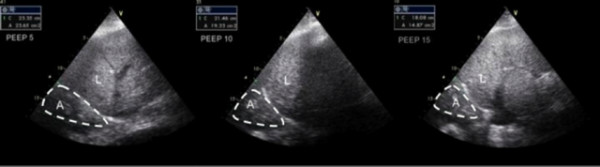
An example of sonographic measurement of the nonaerated lung area at 5, 10 and 15 cm H_2_O positive end-expiratory pressure at the same posterior-axillary line in a patient with acute respiratory distress syndrome. A = nonaerated lung area, L = liver.
